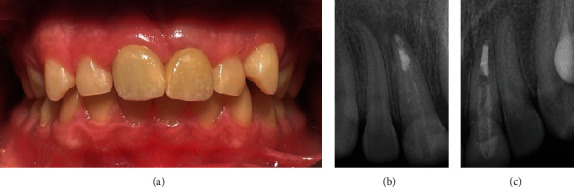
Six-month postsurgery follow-up. (a) Clinical picture showing healthy gingiva and good esthetics. (b, c) PA radiographs showing healing of the surgical site and proper adaptation and proximal contour of the new crowns.
